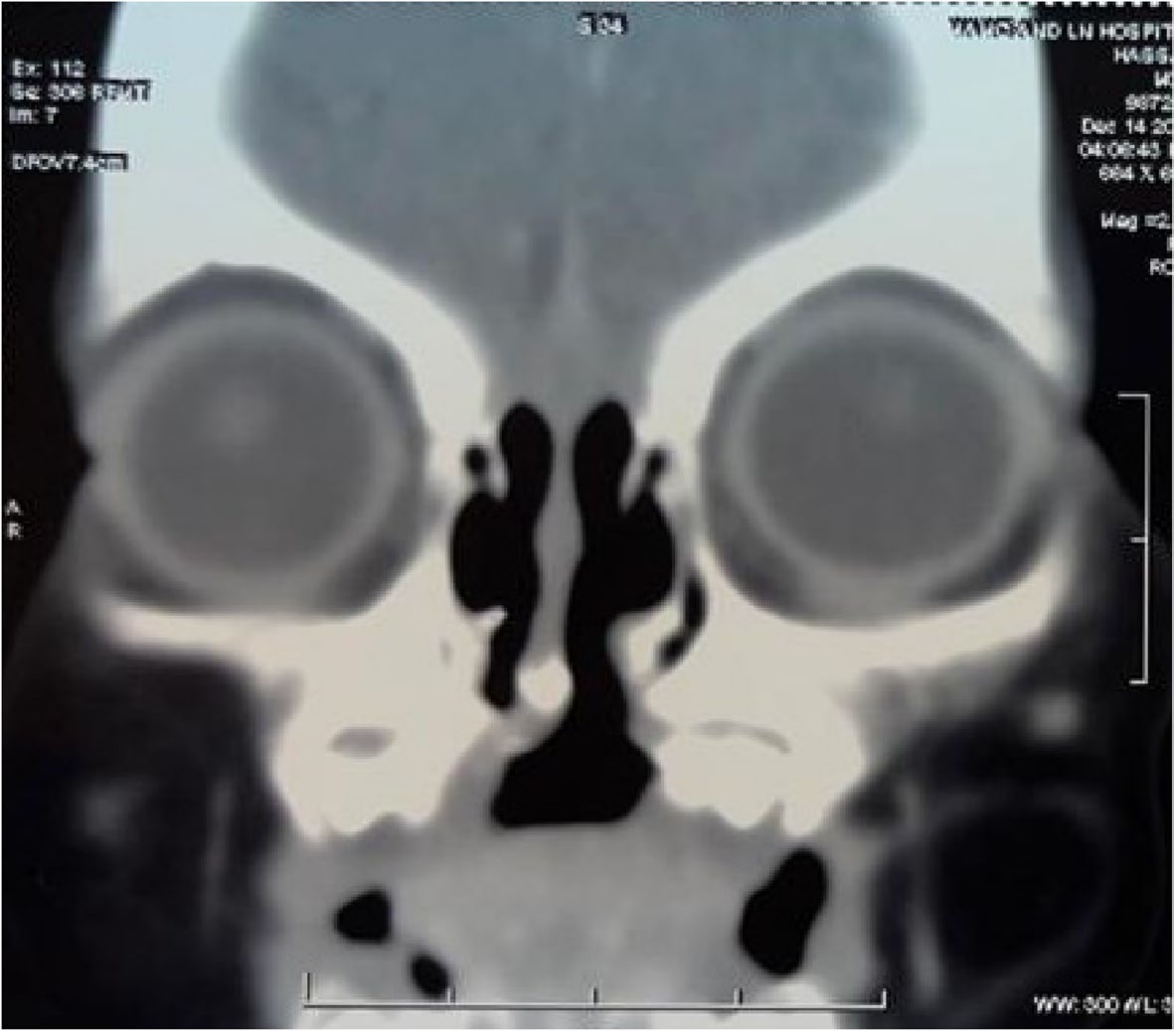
CECT CT face coronal cuts showing the midline palatal defect
